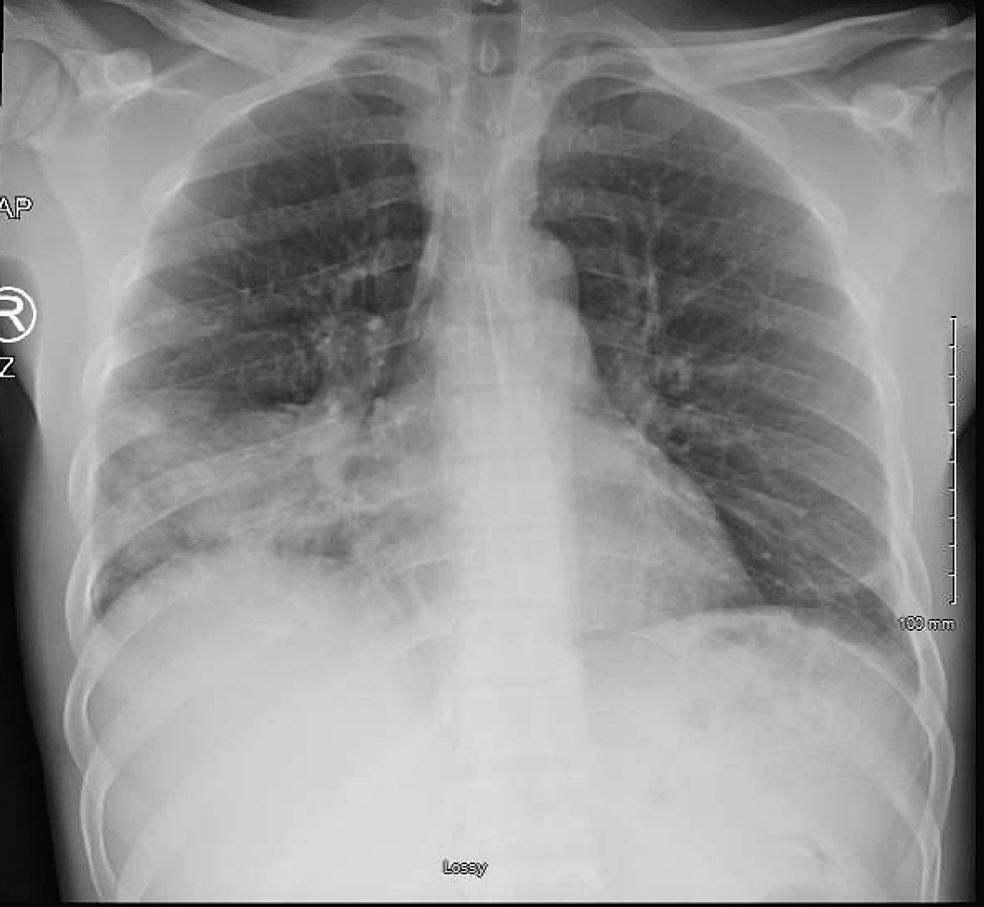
A chest X-ray showing right-sided lower lobe consolidation with an air bronchogram.
